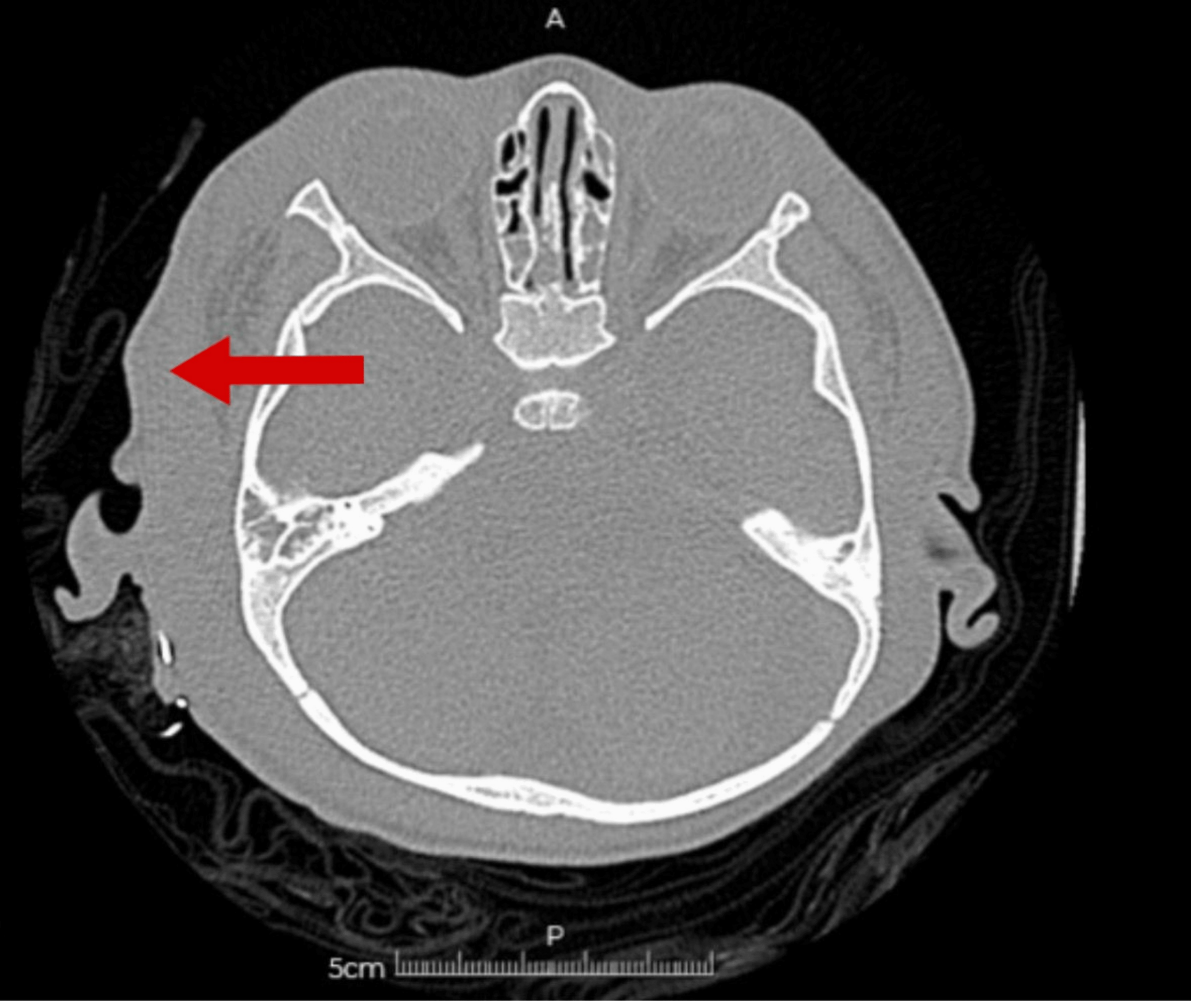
Head CT with an arrow pointing to an area of low attenuation within the subcutaneous tissue at the superolateral aspect of the right periorbital region, with irregular, poorly defined borders, associated with marked edema of the periorbital soft tissues.
